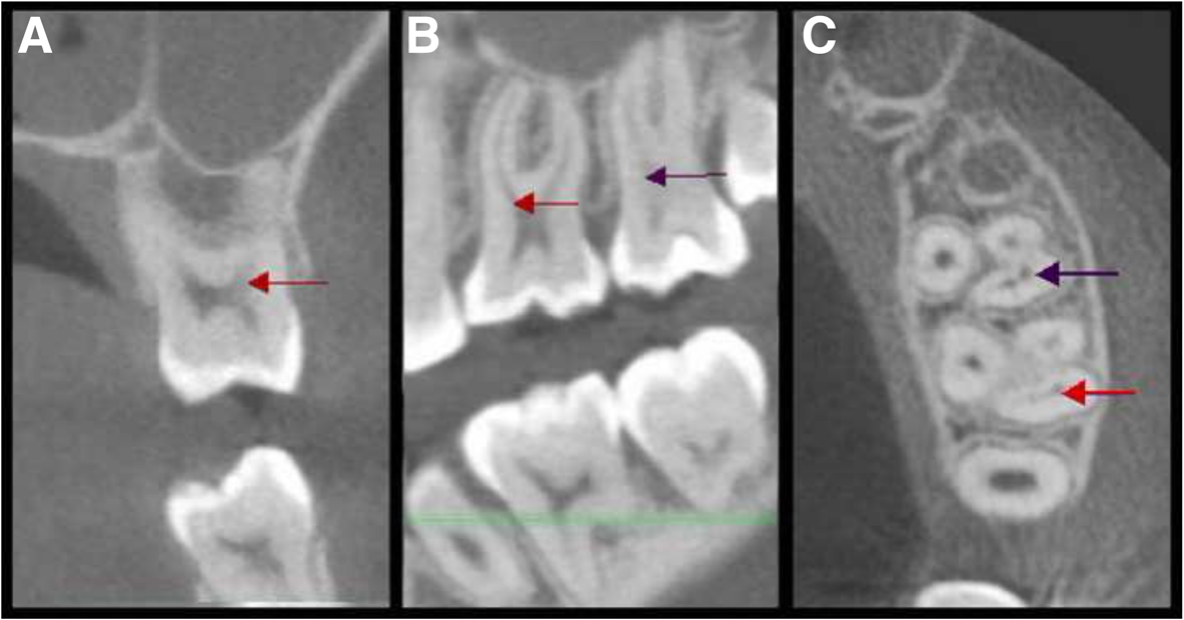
CBCT images of a left maxillary first molar (red arrows) and left maxillary second molar (purple arrows). Mesiobuccal root with 2 canals as viewed in coronal, sagittal, and axial directions using Ez 3D 2009 software. a coronal view; b sagittal view; c axial view
